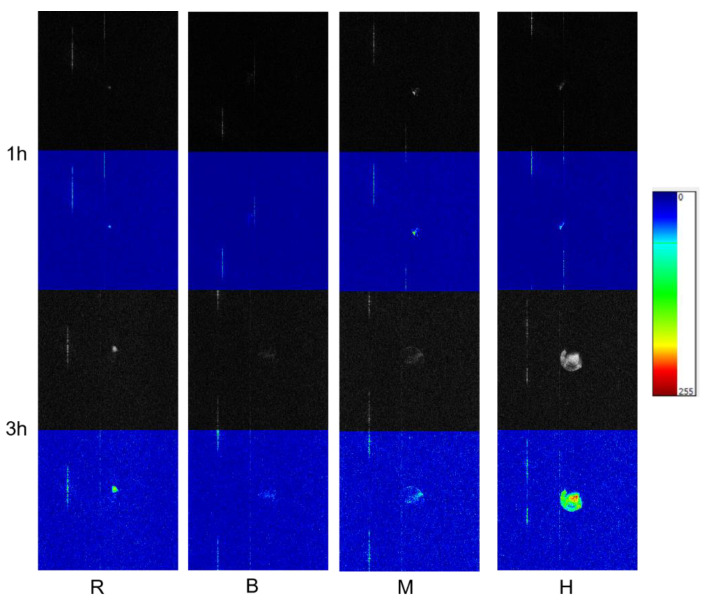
Low-field magnetic resonance imaging of EFS after different thermal processing. R: Raw; M: Microwave; B: Baking; H: Industrial infrared heating-assisted fluidized bed (IHFH).
